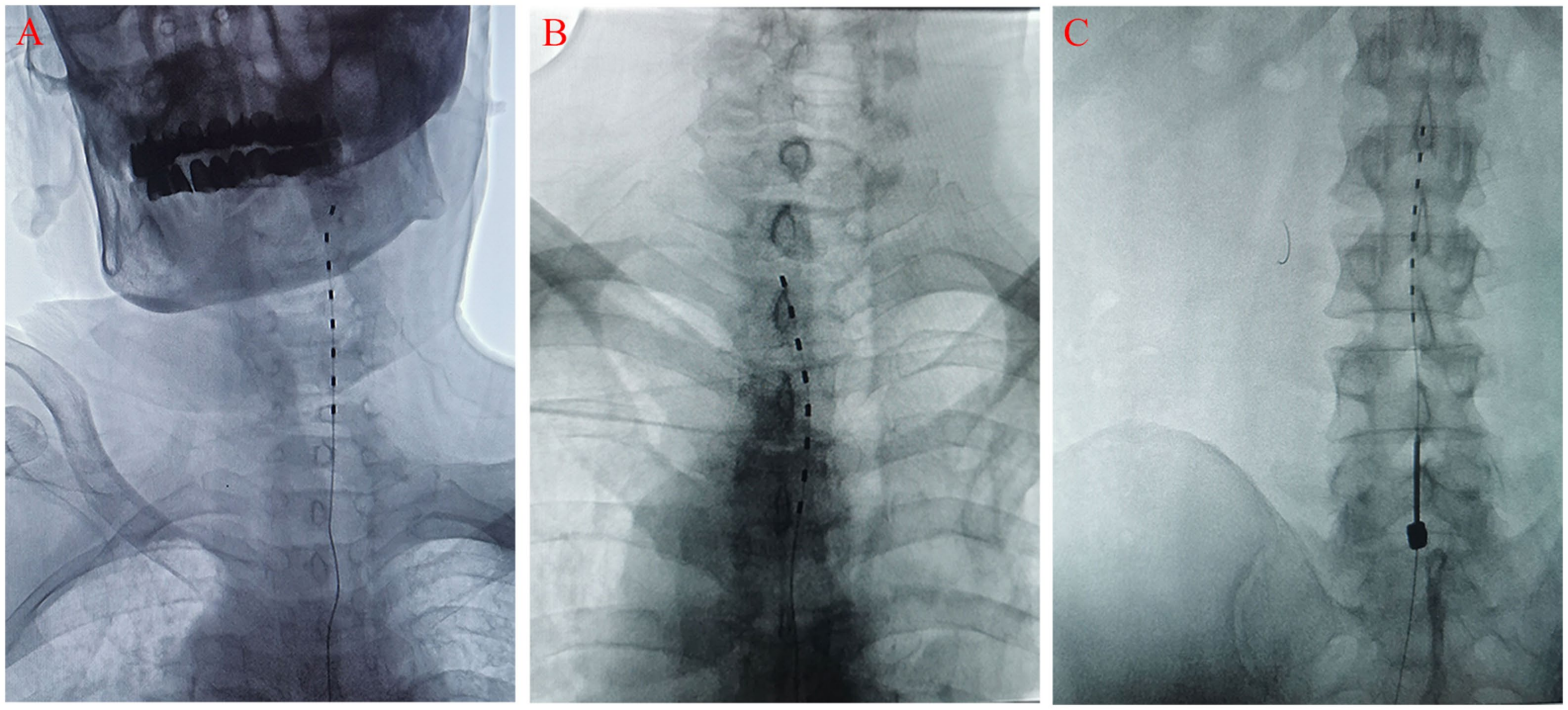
The anteroposterior X-ray film shows that the electrode is located in the target area outside the spinous process and inside the pedicle of vertebral arch. (A) neck; (B) chest; (C) lumbar.
